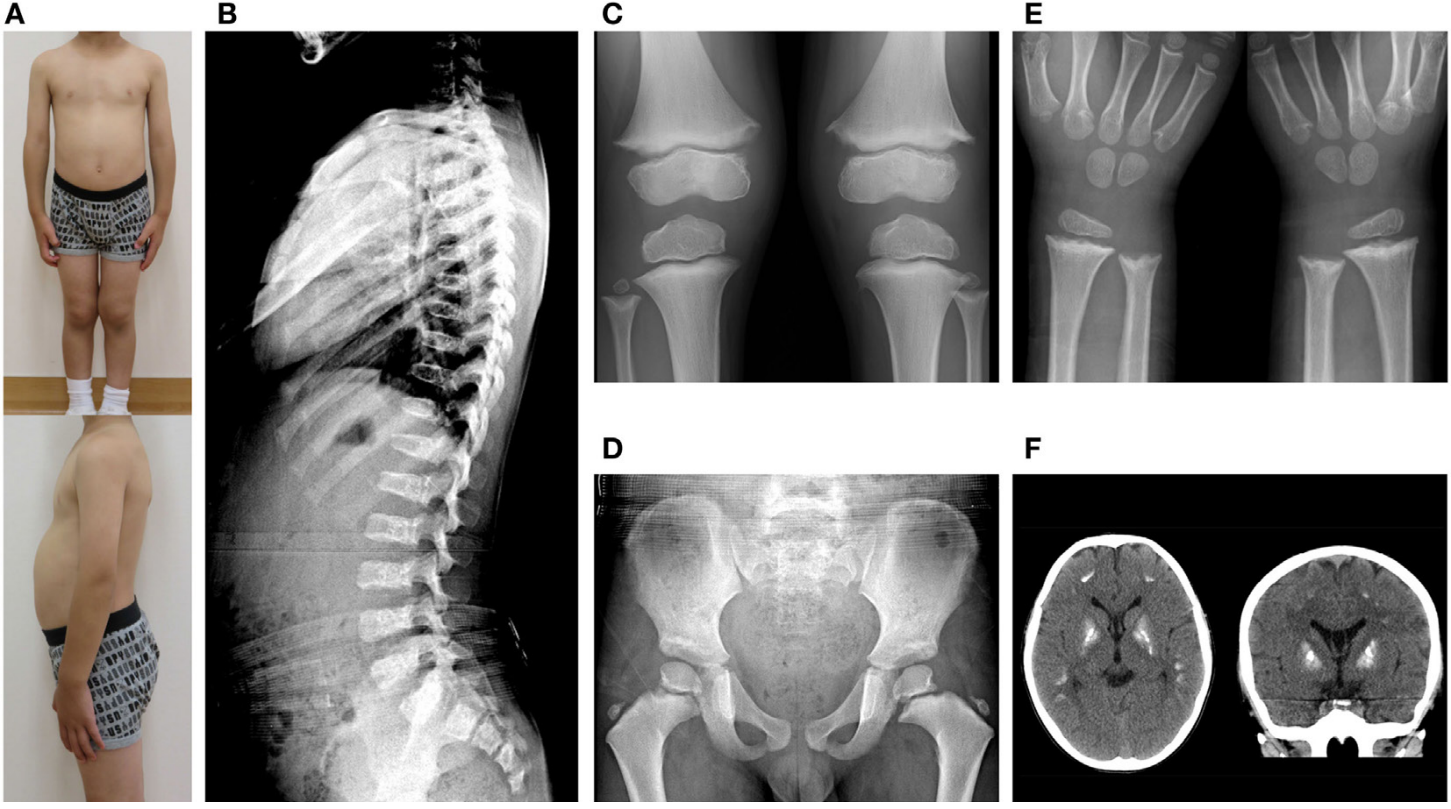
(A) Clinical photographs. (B) Spinal radiograph showing generalized platyspondyly with irregular endplates and irregular ossifications in the posterior vertebral bodies. (C) Radiograph of the knees showing irregular and sclerotic splayed metaphyses of the distal femur, proximal tibia, and fibula. (D,E) Radiograph of the hip and wrist showing similar metaphyseal changes as the knee. (F) Brain CT showing bilateral calcification of the basal ganglia.
